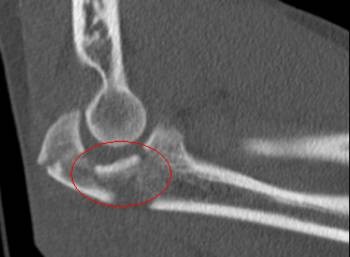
Preoperative diagnostics should include CT imaging to detect an intermediate fragment.
